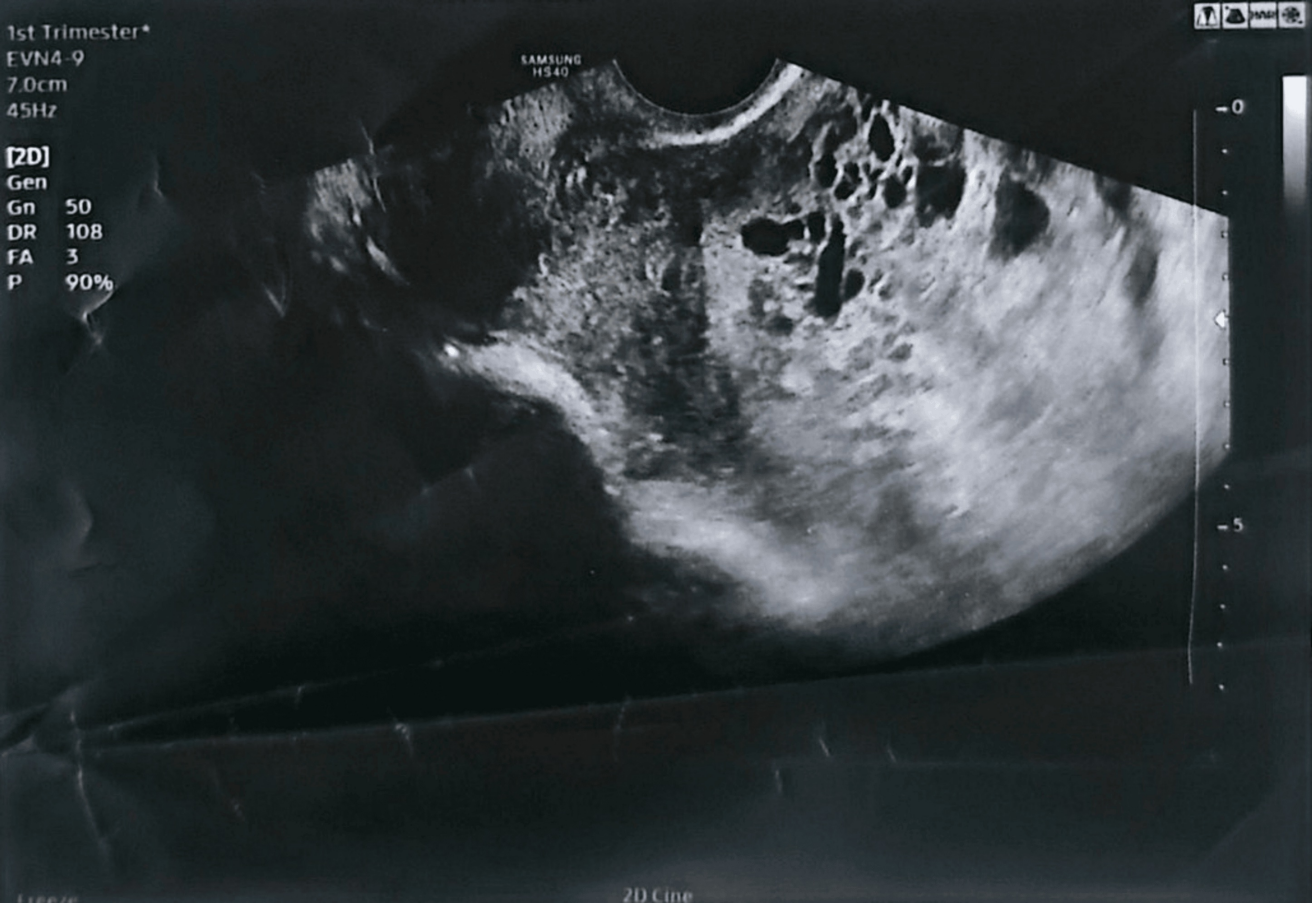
Transvaginal Ultrasound Showing the “Snowstorm” Appearance of a Complete Hydatidiform Mole
